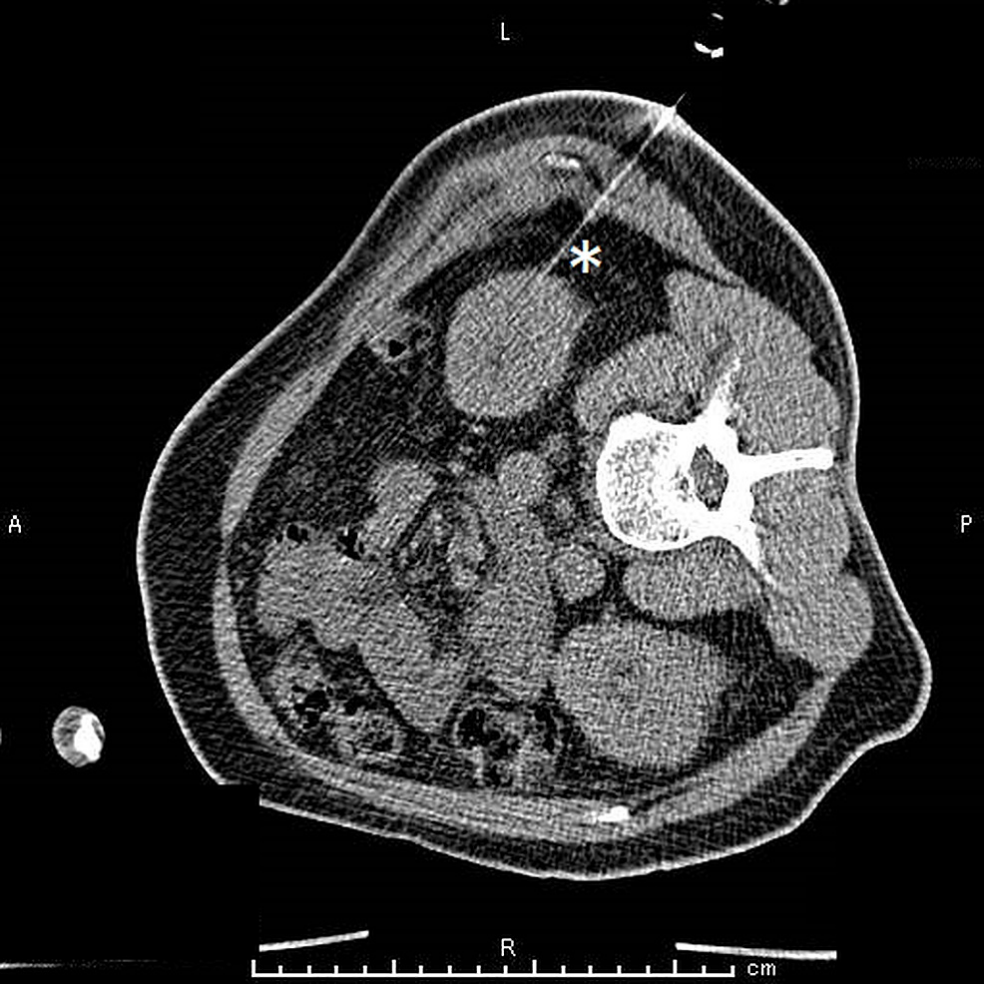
CT-guided kidney biopsy performed using a 17-gauge needle (white asterisk).
